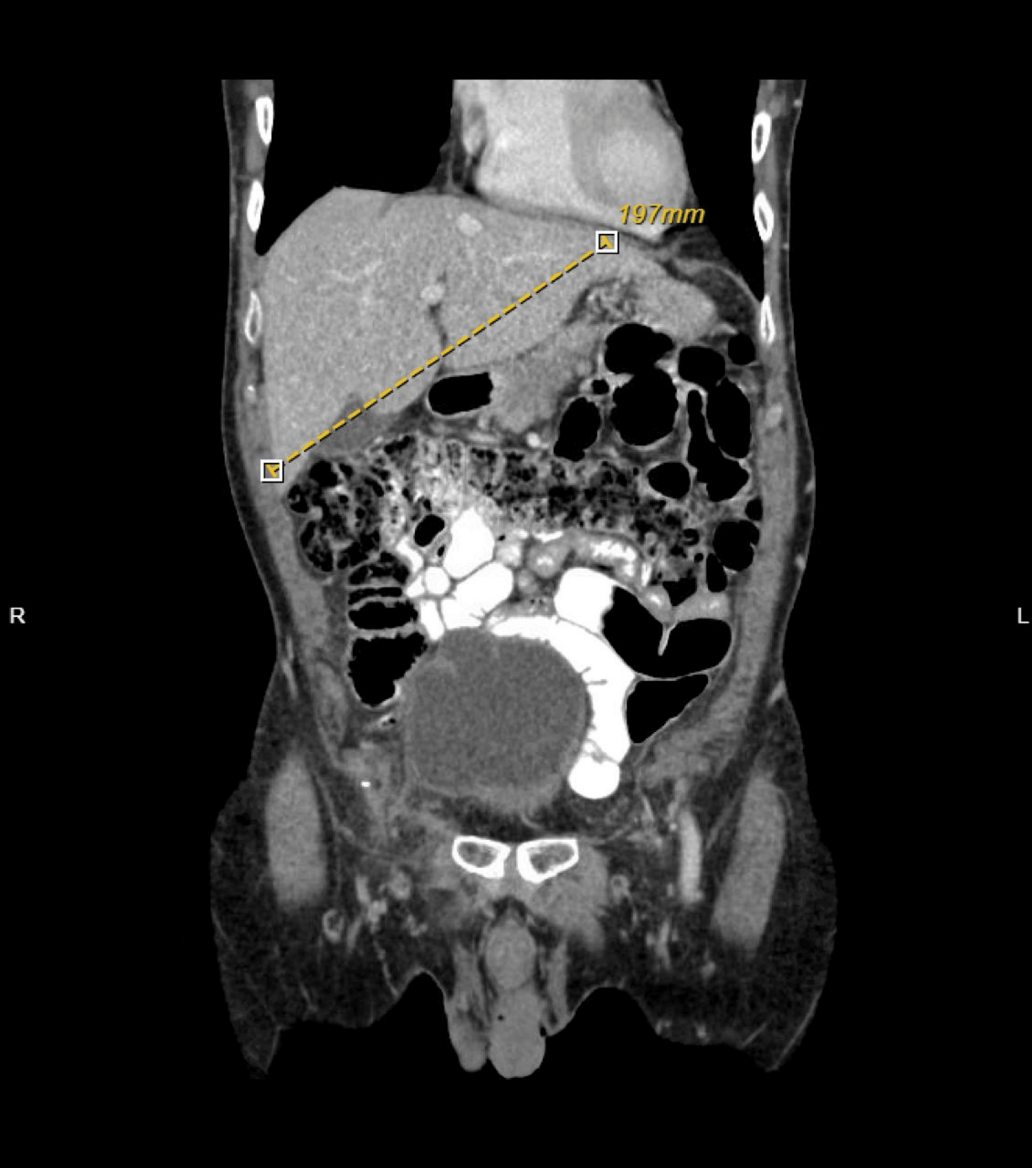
CTAP showing hepatic enlargement. CTAP, computed tomography arterial portography.
